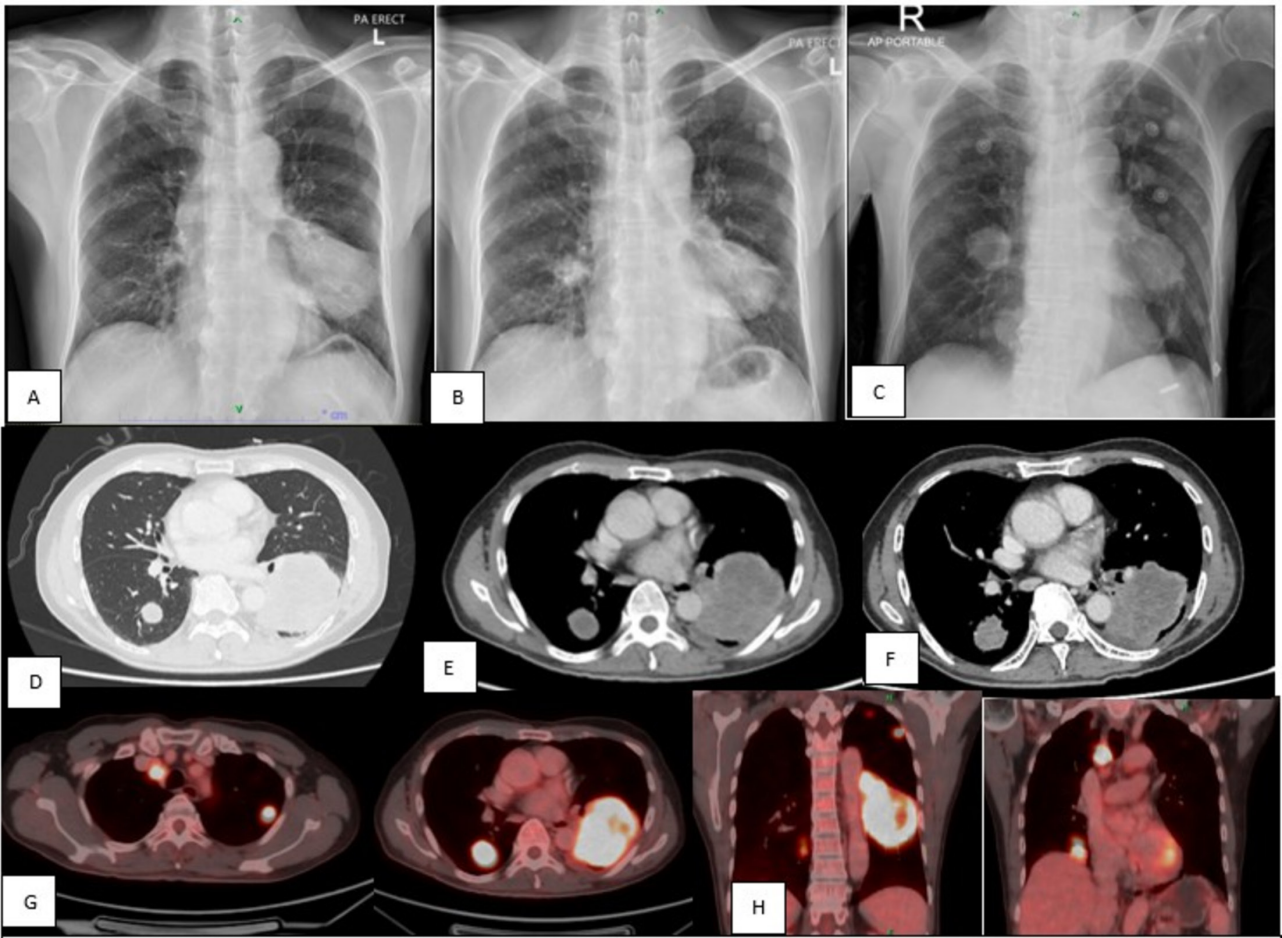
(A–C) Chest radiograph showing left lower zone mass and enlarging mass with multiple nodular opacities in bilateral lungs at diagnosis, 8 weeks and 12 weeks immunotherapy. (D–F) CECT thorax showing an irregular mass at the left lower lobe and a nodule at the left lower lobe with an enlarging mass and multiple nodular metastases at diagnosis, 8 and 12 weeks of immunotherapy. G and H showed PET CT at 12 weeks post‐immunotherapy.
